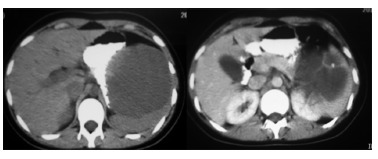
CT scan showed a giant cyst which squeeze the stomach
